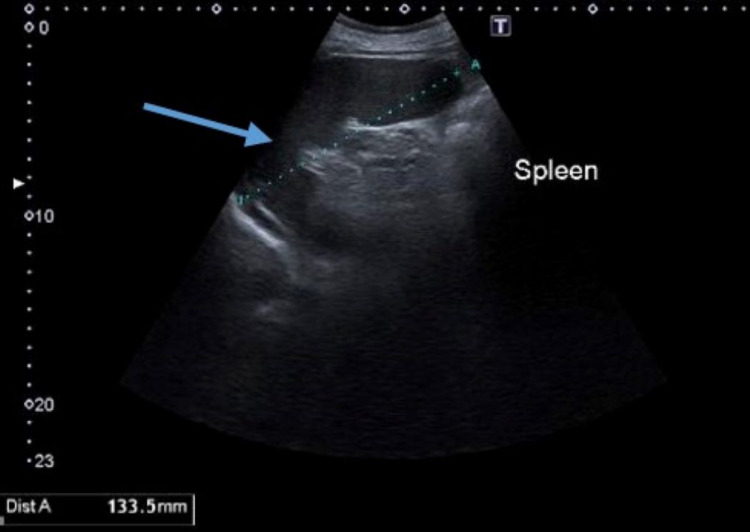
Ultrasound of abdomen Ultrasonography of the patient's abdomen revealed splenomegaly. The blue arrow is pointing towards the spleen. The dotted line shows the entire extent of the spleen.
